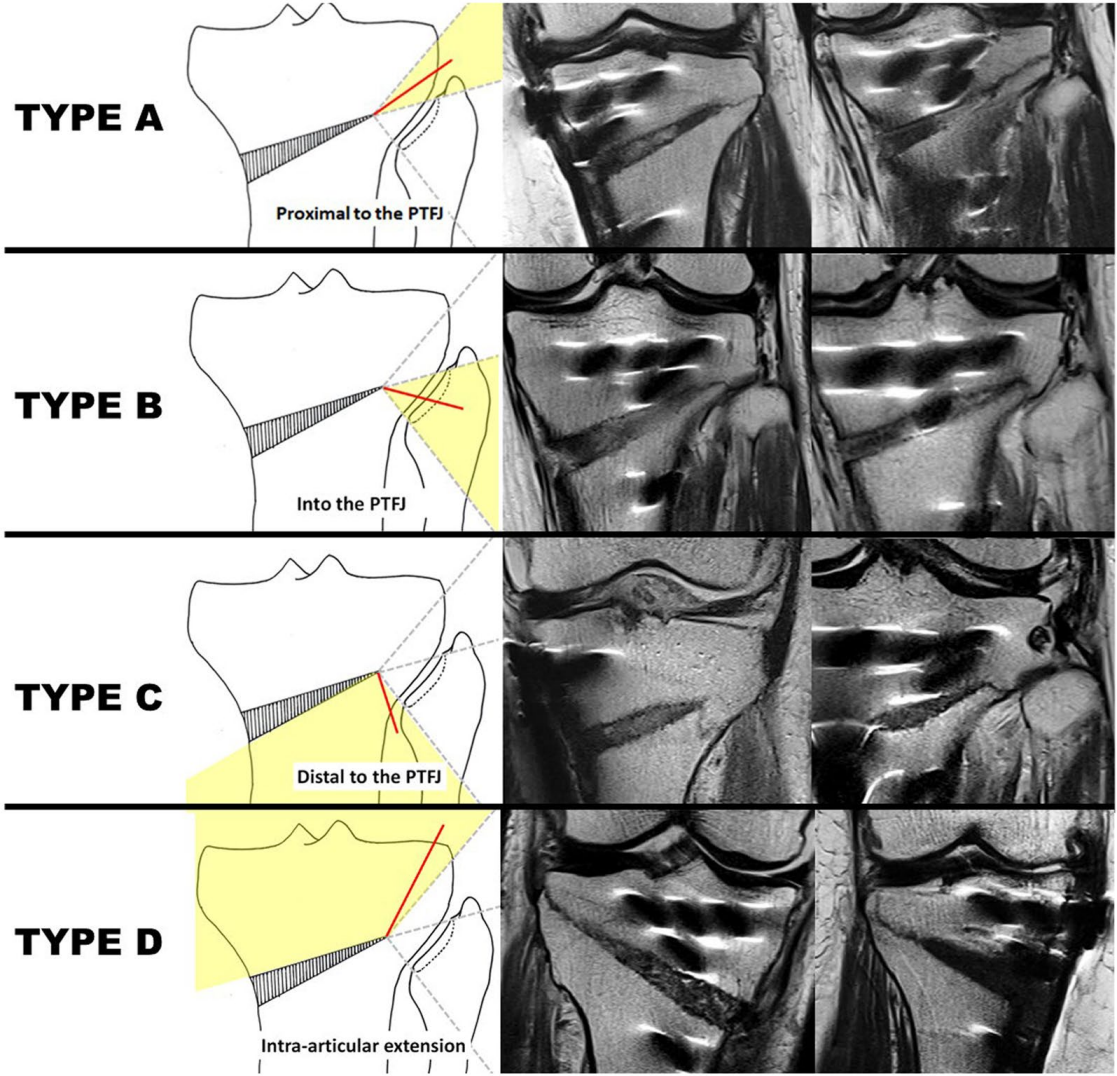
Examples of MRI-based classification (coronal cut sections). The red lines indicate the fracture line. In type A, the fracture occurs where the osteotomy line extends proximally to the tibiofibular joint without involving the joint itself. Type B refers to a fracture line that extends into the proximal tibiofibular joint. In type C, the fracture extends distal to the proximal tibiofibular joint. Finally, type D is characterized by a fracture that extends proximally into the joint
